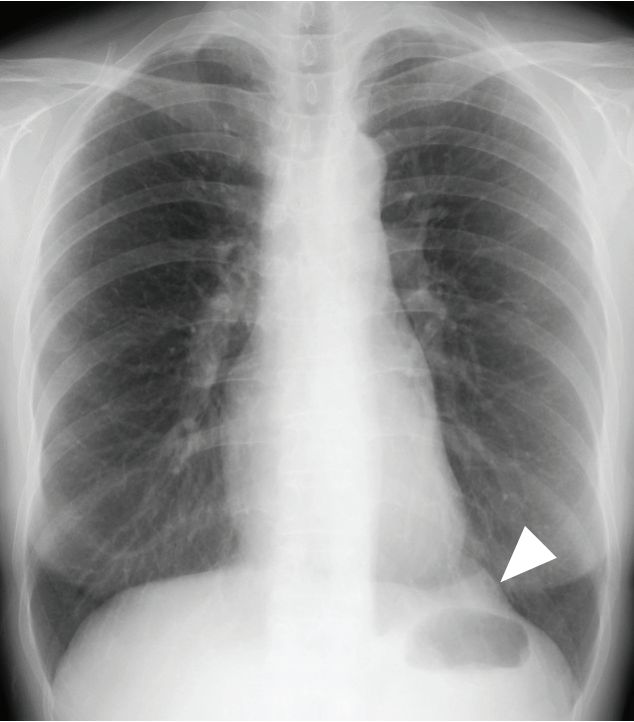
Chest radiograph showing a nodule along the pericardium toward the left hemidiaphragm (arrowhead).
